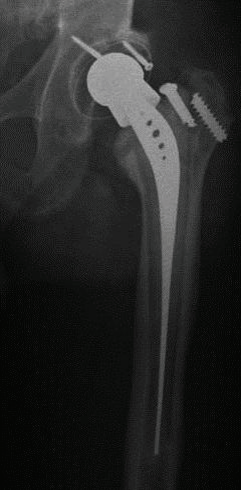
Twenty years after primary hip arthroplasty. The radiograph shows loosening of the isoelastic cementless femoral stem and well-fixed titanium-coated RM acetabular cup.
